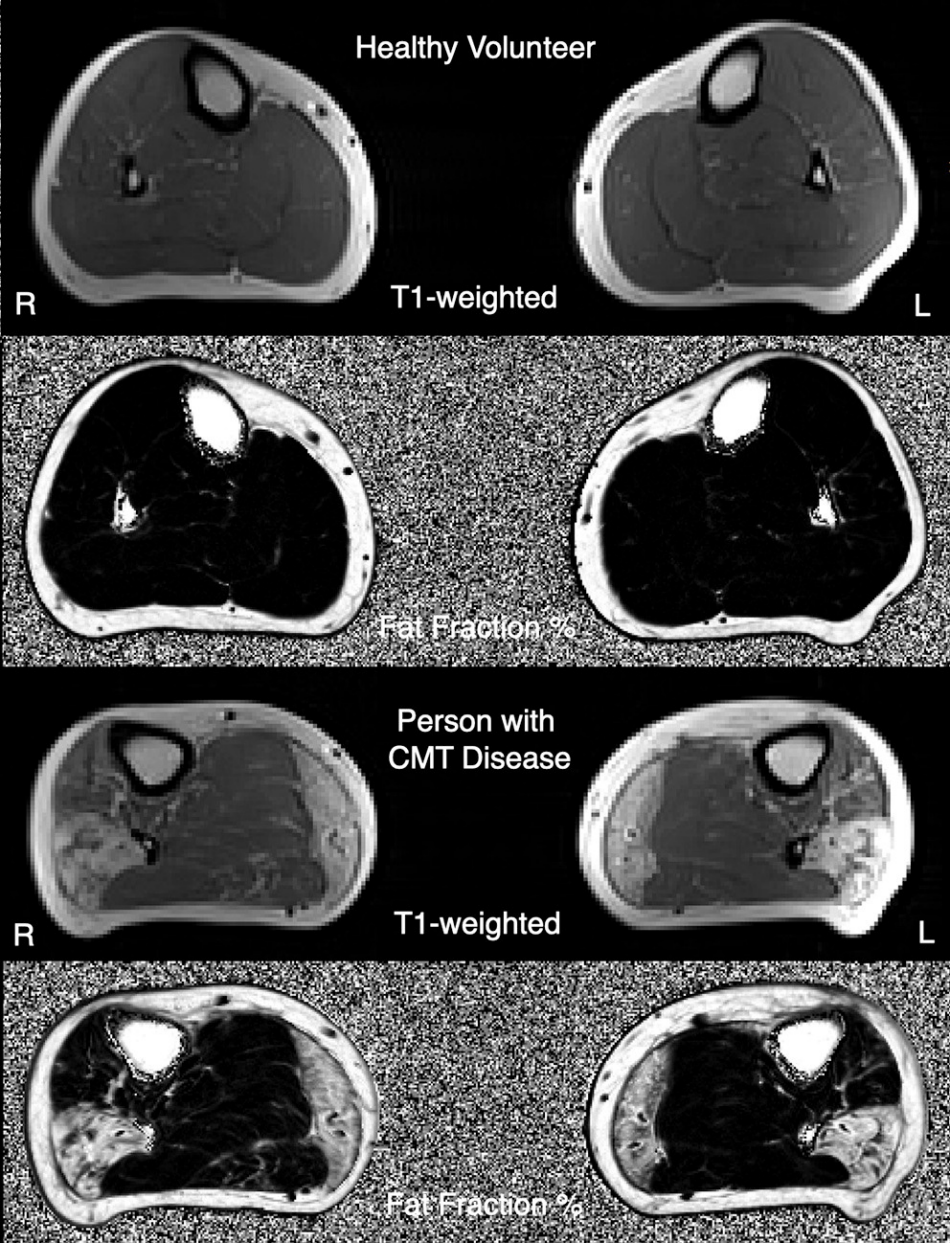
Example T1-weighted MRI images, and three-point Dixon MRI-obtained quantitative fat-fraction maps, from a healthy volunteer (top images), and a person with CMT1A (bottom images).
